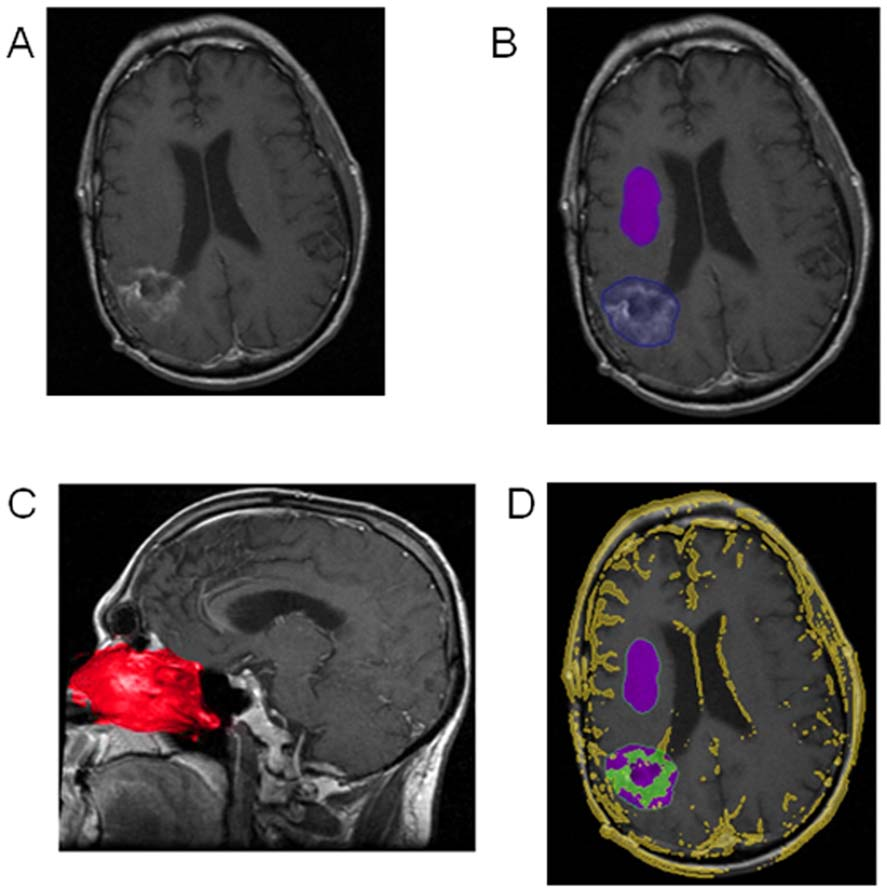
Automated Assessment of Enhancing Tumor Volume. A) T1-weighted post-contrast axial images are automatically fused with the pre-contrast sequences. B) The tumor region of interest (blue area) and nearby normal brain (purple area) are outlined roughly by hand. C) The enhancing nasal mucosa region is automatically detected with a built-in anatomic atlas (red area) and serves as a threshold for enhancement. D) Tissue that is present on the post-contrast images but not the pre-contrast that is above the enhancement threshold appears in yellow. This includes enhancing tissue such as vasculature, tumor, and superficial structures. Enhancing tumor volume is defined as the green area within the manually-defined blue tumor region of interest.
